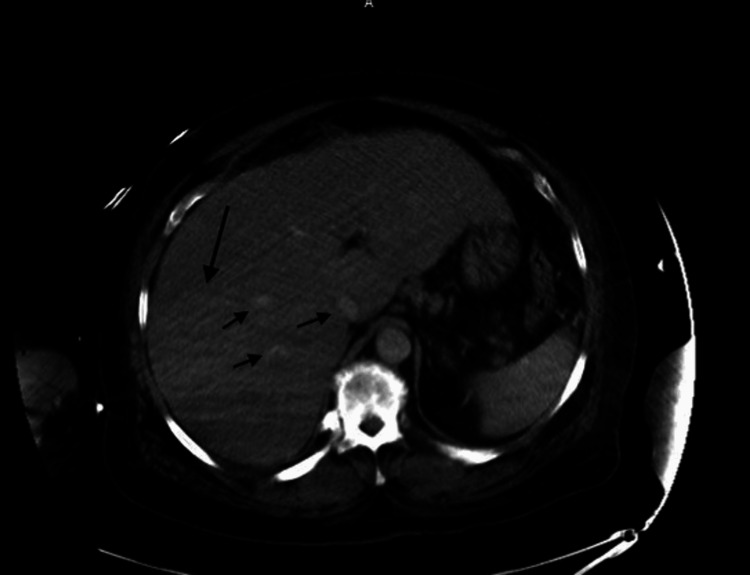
CT of the abdomen and pelvis showing hepatomegaly and heterogeneous parenchyma concerning for cirrhosis or metastatic lesions
